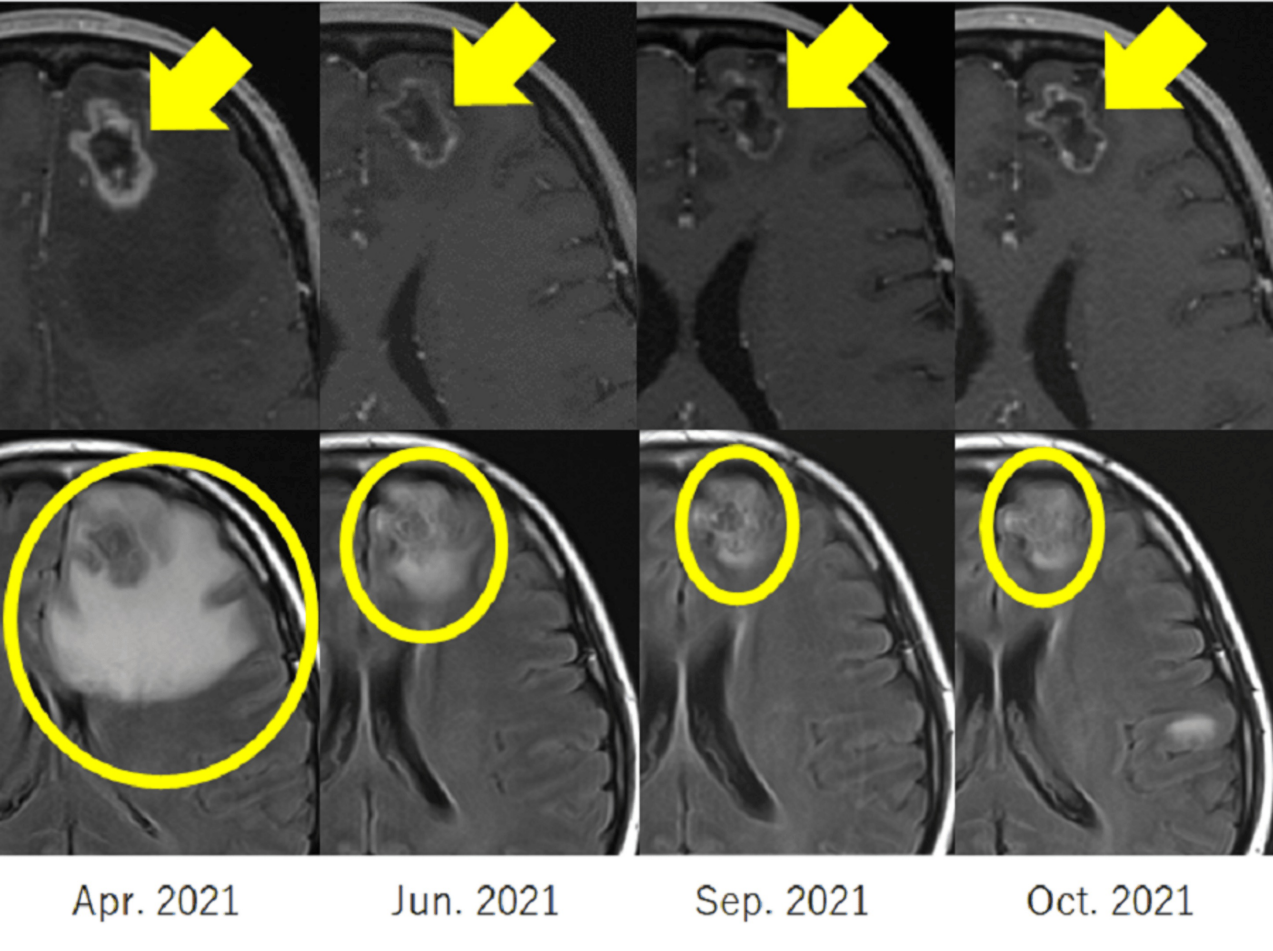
Course of brain necrosis upper: gadolinium-enhanced T1-weighted imaging (Gd-T1WI), lower: fluid-attenuated inversion recovery (FLAIR)
